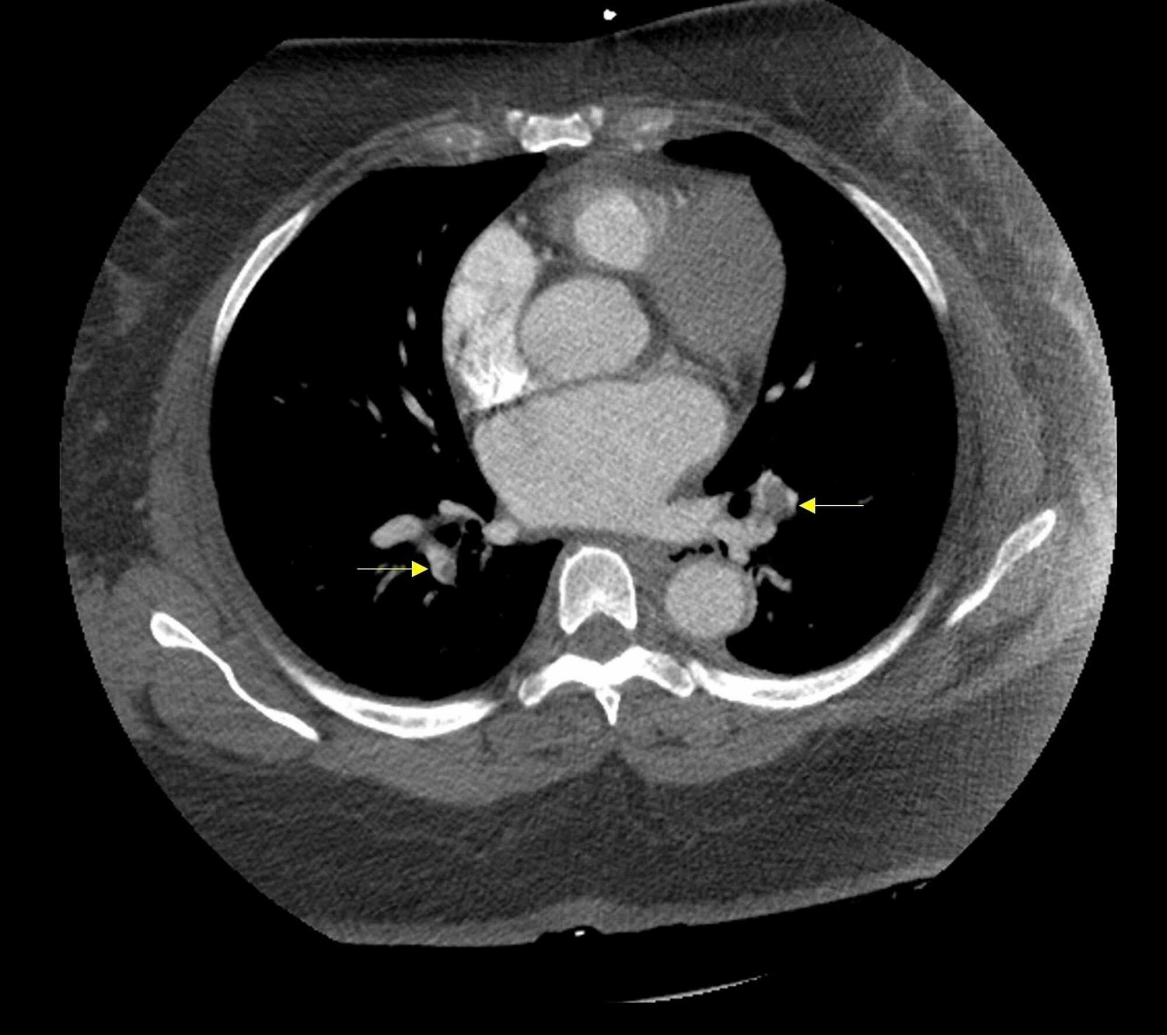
Computed tomography angiography of the chest showing bilateral pulmonary embolism (yellow arrows).
